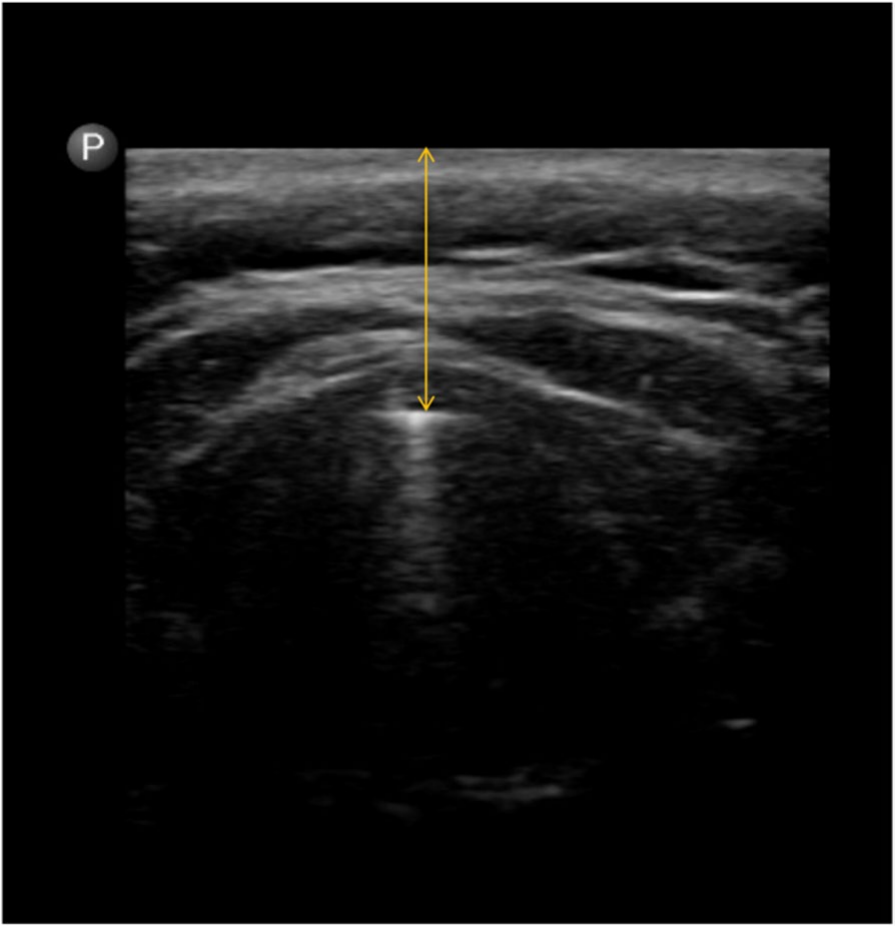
Transverse ultrasound view of the distance between skin and epiglottis at the vocal cord level (arrow)
